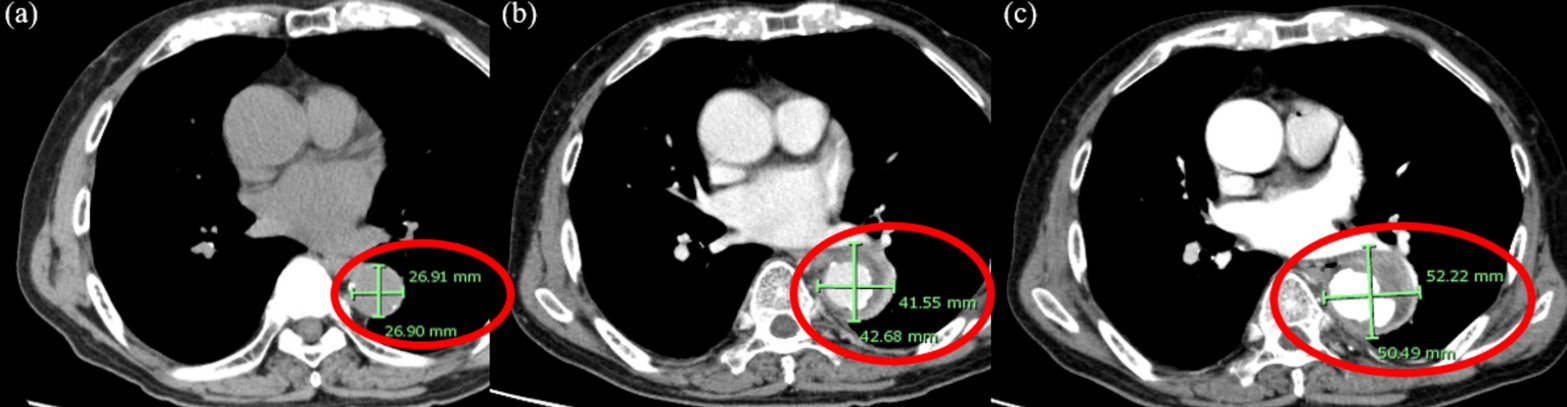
The horizontal slice of chest CT (a) The aortic diameter was 26.9 mm x 26.9 mm on eight days before admission(red round). It also showed calcification of the aortic wall. (b) The aortic diameter was 41.5 mm x 42.6 mm on the day of admission (red round). (c) The aortic diameter was 52.2 mm x 50.4 mm on day 6 after admission (red round), indicating an increase in aortic diameter with time.
